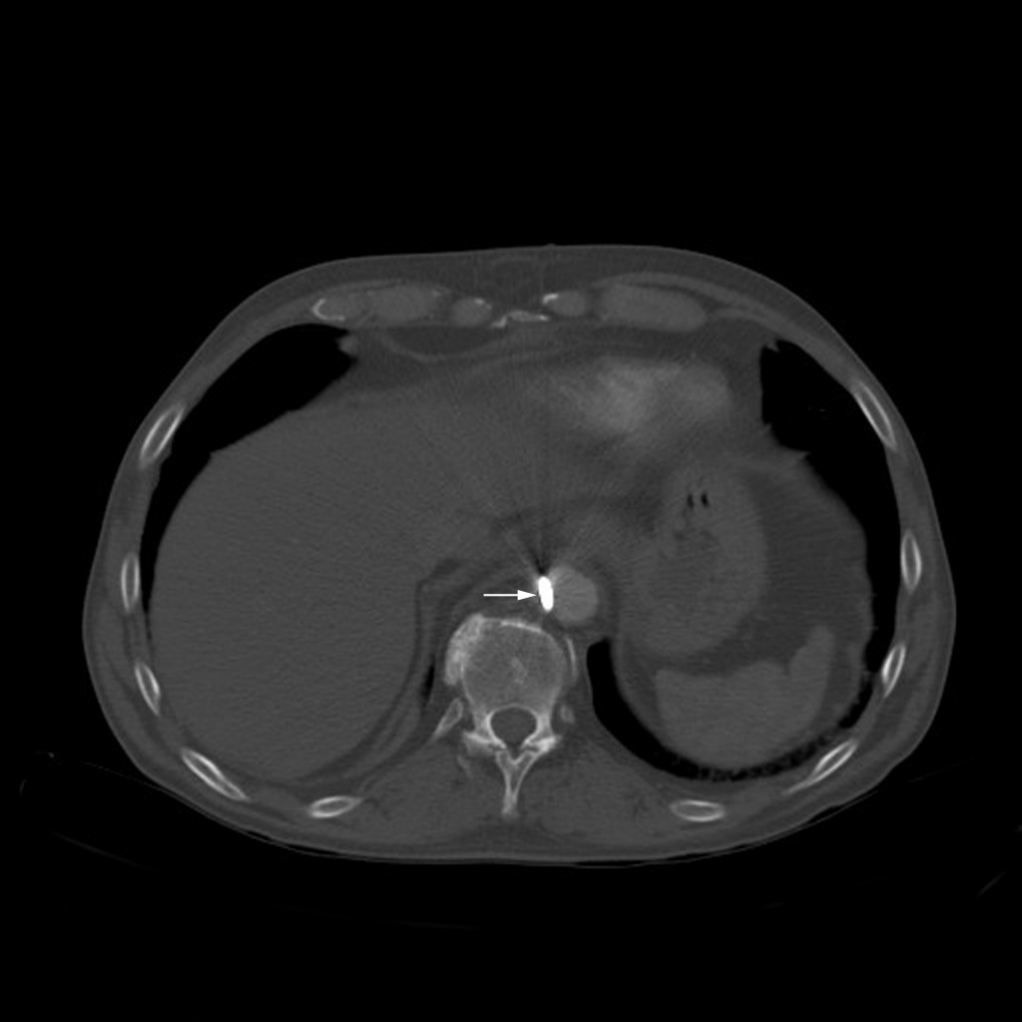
Axial contrast-enhanced computed tomography (CT) Axial contrast-enhanced computed tomography (CT) image of the abdomen showing nail tip adjacent to the abdominal aorta (arrow).
